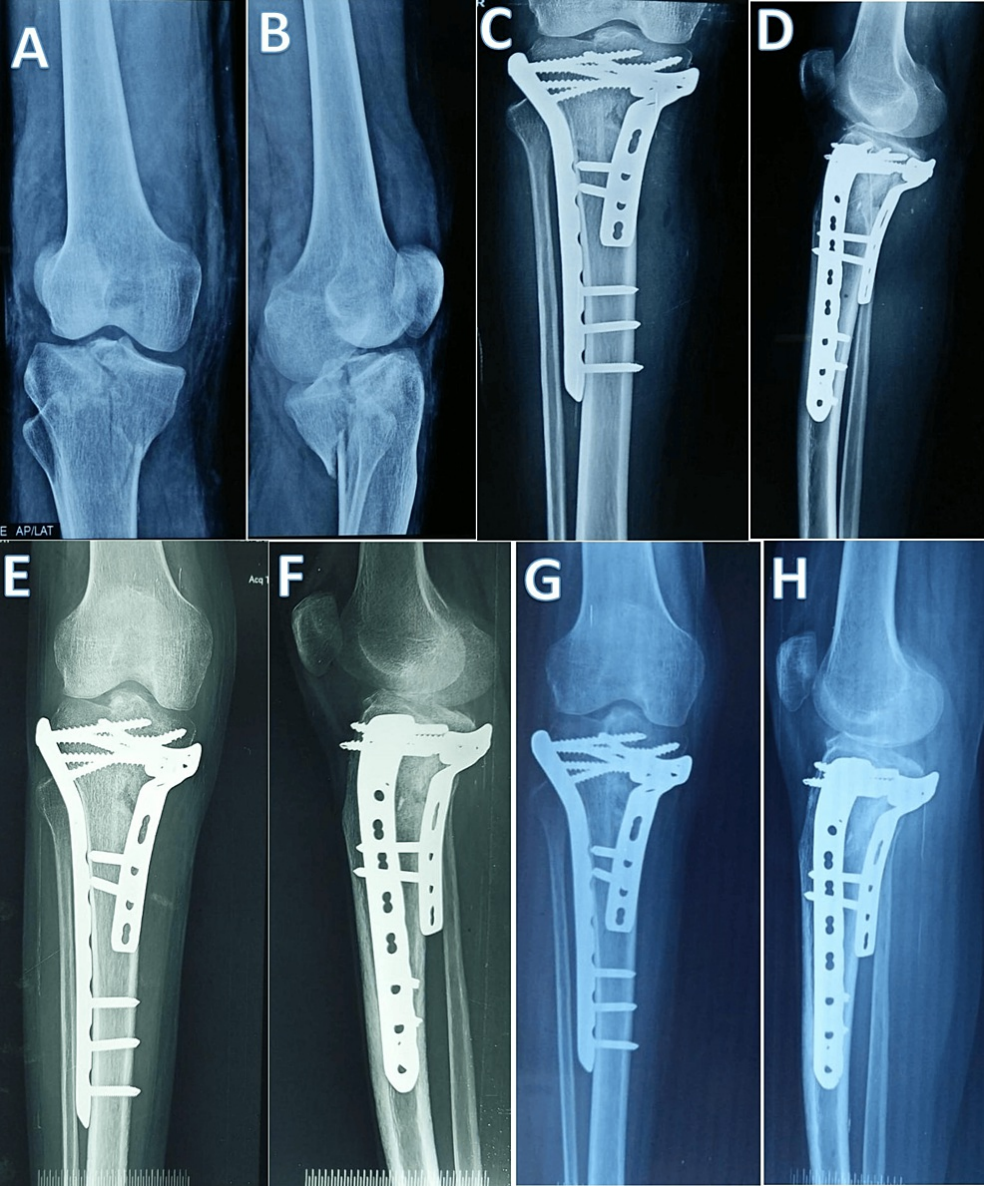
Follow-up radiographs of a 33-year-old male patient with a complex unstable right proximal tibial plateau fracture that was fixed with two plates. (A and B) Preoperative radiographs showing the proximal tibial plateau fracture pattern. (C and D) Immediate postoperative radiograph of the fracture fixed by dual plating (anterolateral plate and posteromedial plate). (E and F) Follow-up radiograph six months after surgery. (G and H) Follow-up radiograph 12 months after surgery.
